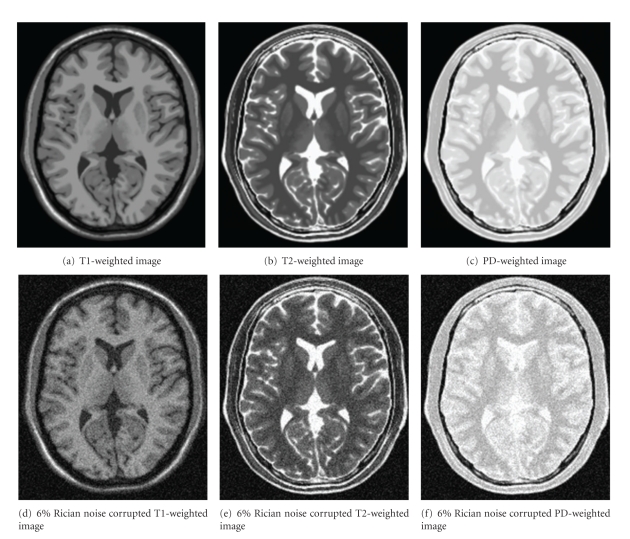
Samples of MR images with Rician noise. (a) to (c) are original images from the BrainWeb Database, (d)–(f) are noisy images of (a)–(c) with 6% Rician noise added.
